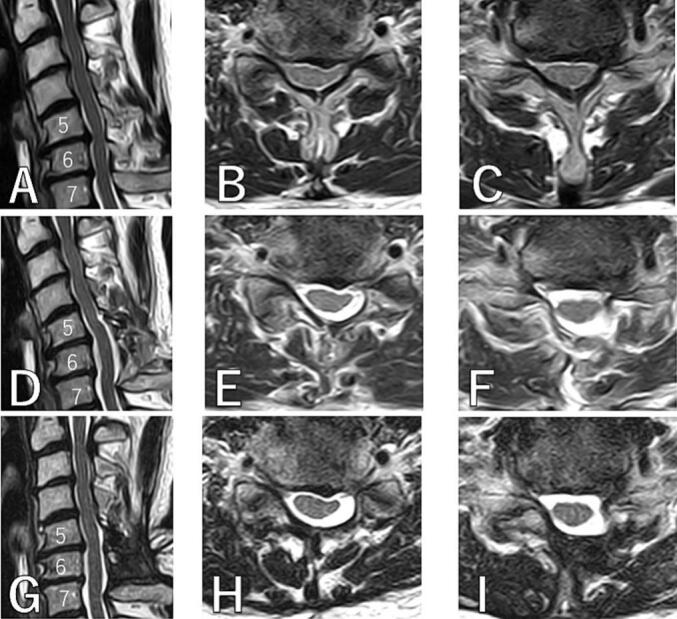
MR images. A, B, C: preoperative. D, E, F: postoperative. G, H, I: 2 years postoperative. A, D, G: sagittal. B, E, H: axial at C5–6. C, F, I: axial at C6–7.
